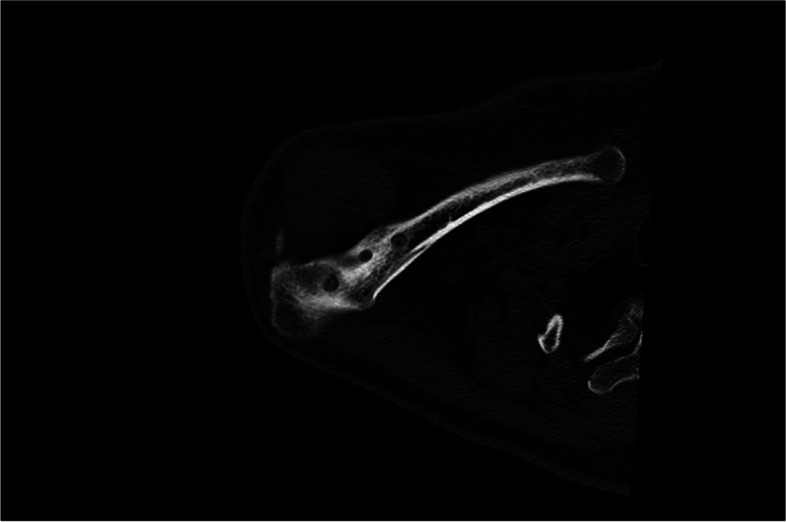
Computed tomography allowing for adequate visualization of bony imparities such as clavicular bone tunnel position, tunnel width or fractures
